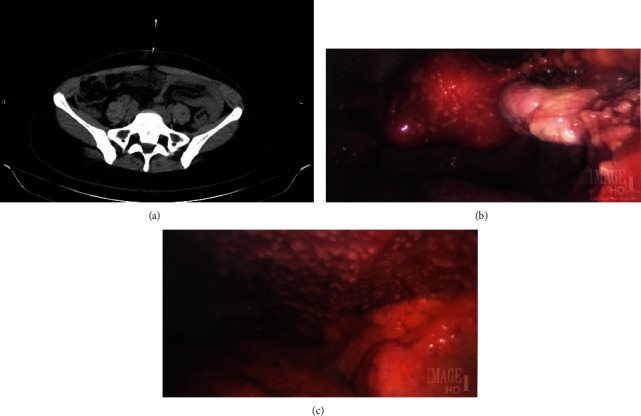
(a) Computed tomography (CT) imaging of the pelvis at the time of presentation and needle biopsy of omental caking. (b) Diagnostic laparoscopy showing innumerable tan, 1-2 mm nodules on the surface of the right ovary and uterus. (c) Diagnostic laparoscopy showing innumerable tan, 1-2 mm nodules on the anterior abdominal wall.
